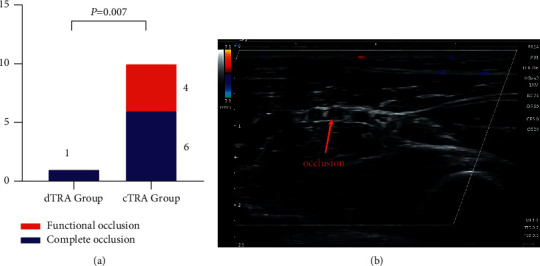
(a) Results of ultrasound follow-up. (b) Ultrasound image of complete radial artery occlusion.
